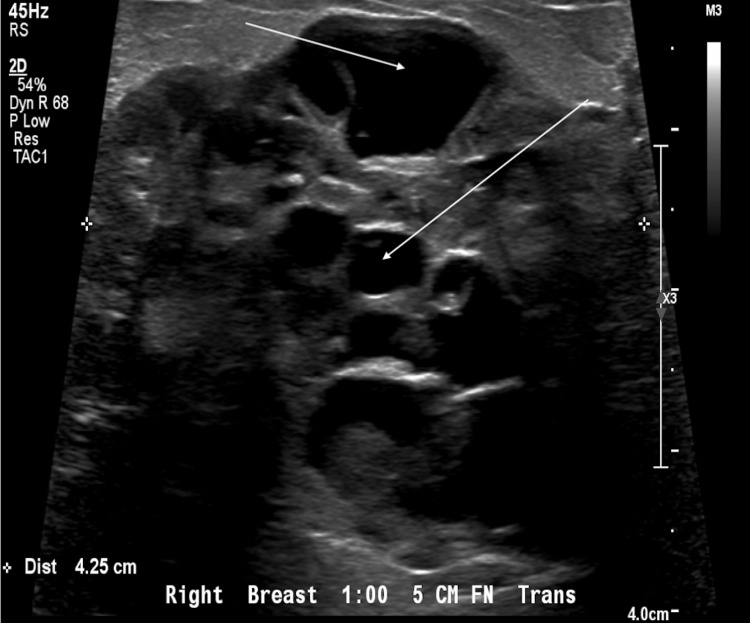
Ultrasound - transverse view. Arrows pointing to the cystic spaces.
